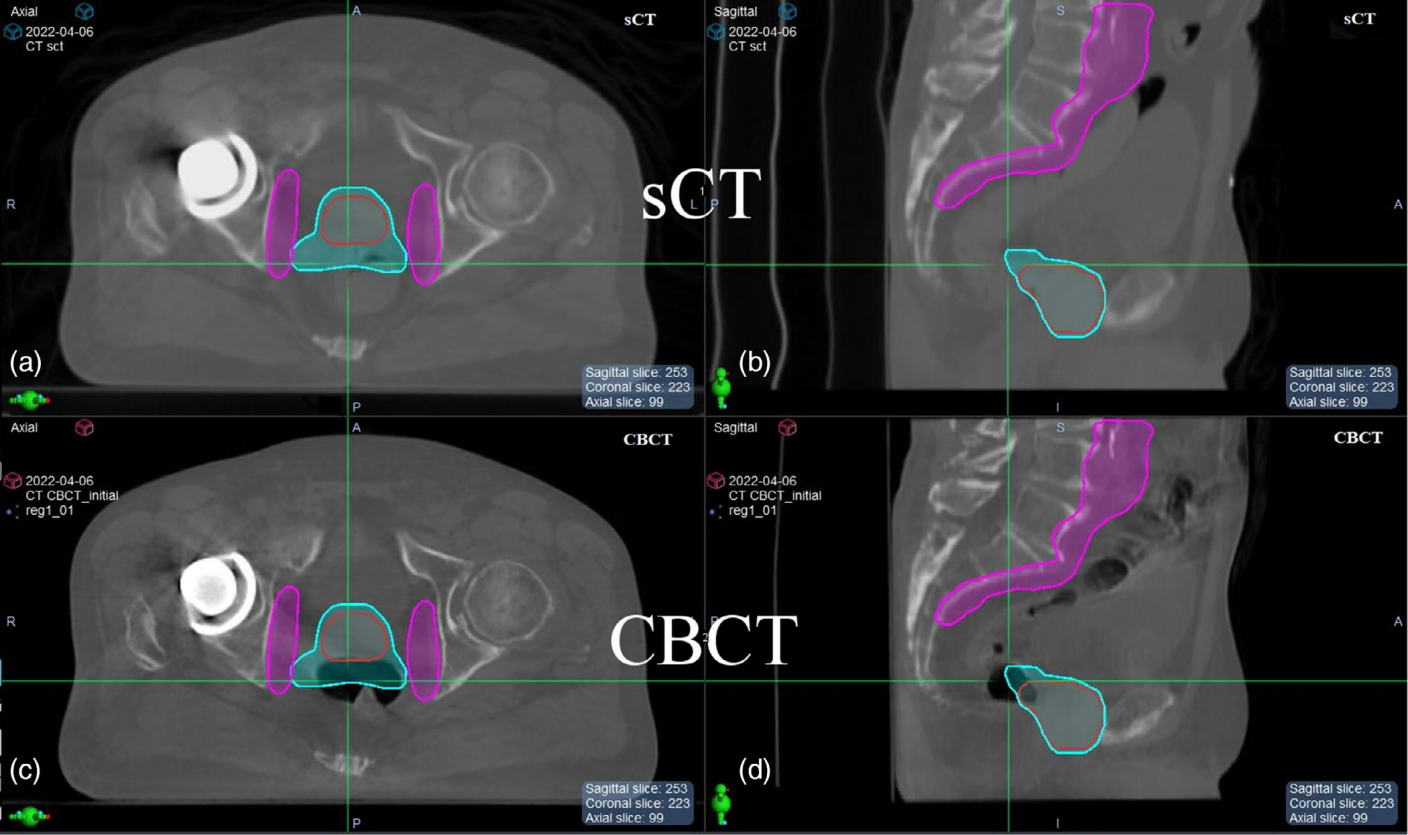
CBCT and respective sCT images for P3‐FX21 showing the differences in abdominopelvic air cavities. (a) sCT axial. (b) sCT sagittal. (c) CBCT axial. (d) CBCT sagittal. PTV4860 (magenta), PTV5400 (cyan), and PTV7800 (red) were overlaid on both images to highlight the proximity and overlap with the uncorrected air volume.
